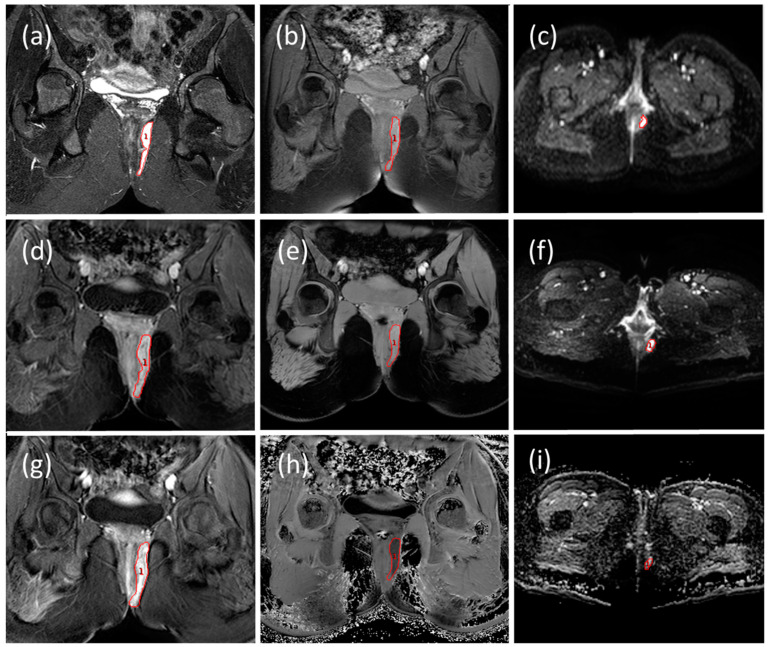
Patient with clearly visible fistula. (a) 1.5 T coronal STIR image where a radiologist drew the ROI and (b) 1.5 T MT ‘on’ image. (c) 1.5 T DWI b0 image shows where the ROI were copied. (d) 3.0 T coronal T1 post-contrast image where a radiologist drew the ROI and (e) 3.0 T MT ‘on’ image. (f) 3.0 T DWI b0 image where the ROI was copied. (g) 3.0T DCE image (h) 3.0 T MTR MAP and (i) 3.0 T ADC MAP with ROI shown from where results were calculated.
